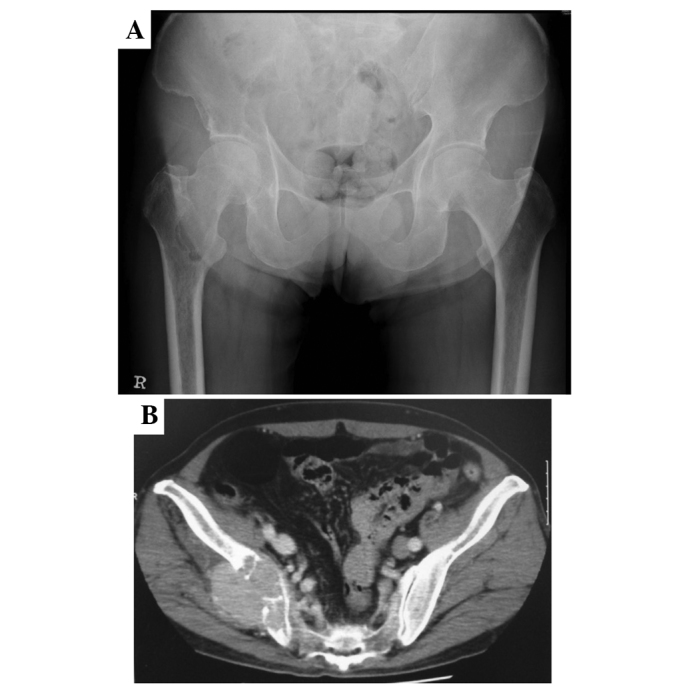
(A) Plain film showing an osteolytic lesion in the right proximal femur. (B) Pelvic computed tomography of a tumor lesion in the right ilium.
